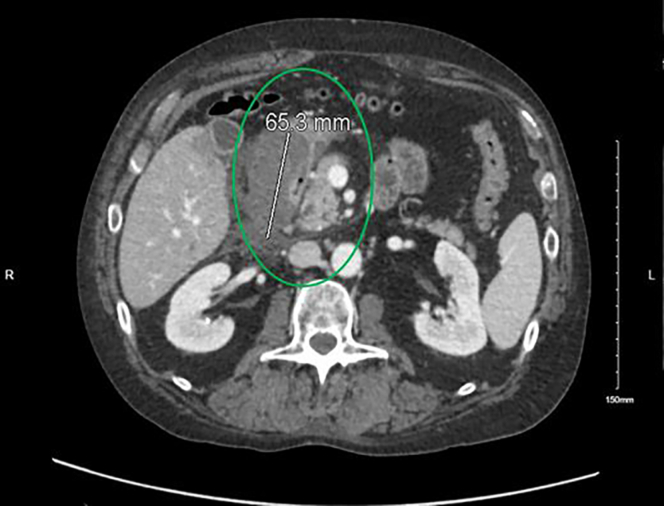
Computed tomography angiography axial view of the intramural duodenal hematoma *(green circle)*.
